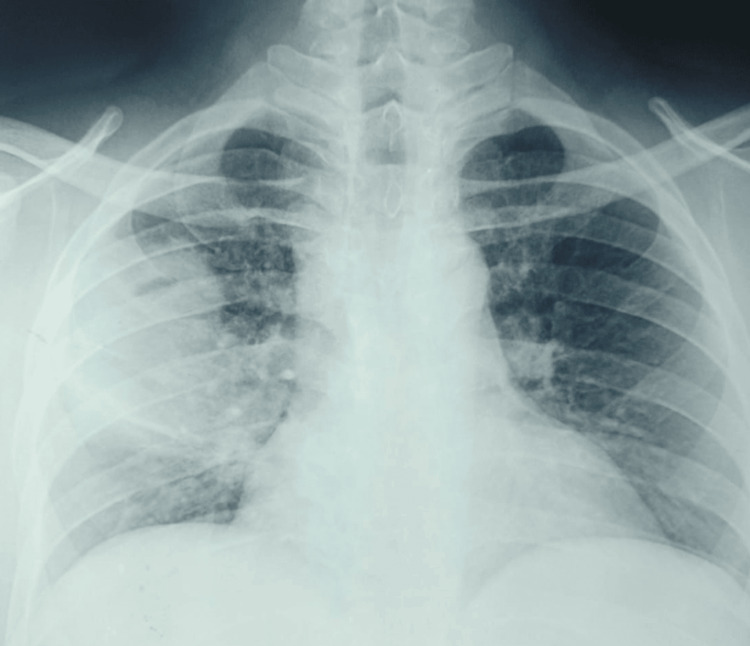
Chest radiograph showing radiological worsening
